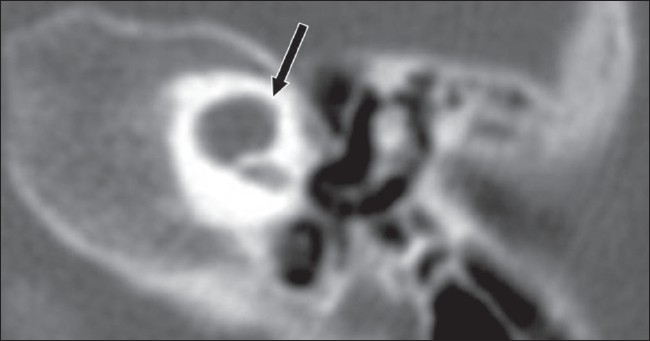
Common cavity. Axial HRCT image shows a common cavity (arrow), in which there is no differentiation between the cochlea and vestibule with a resultant cystic cavity
